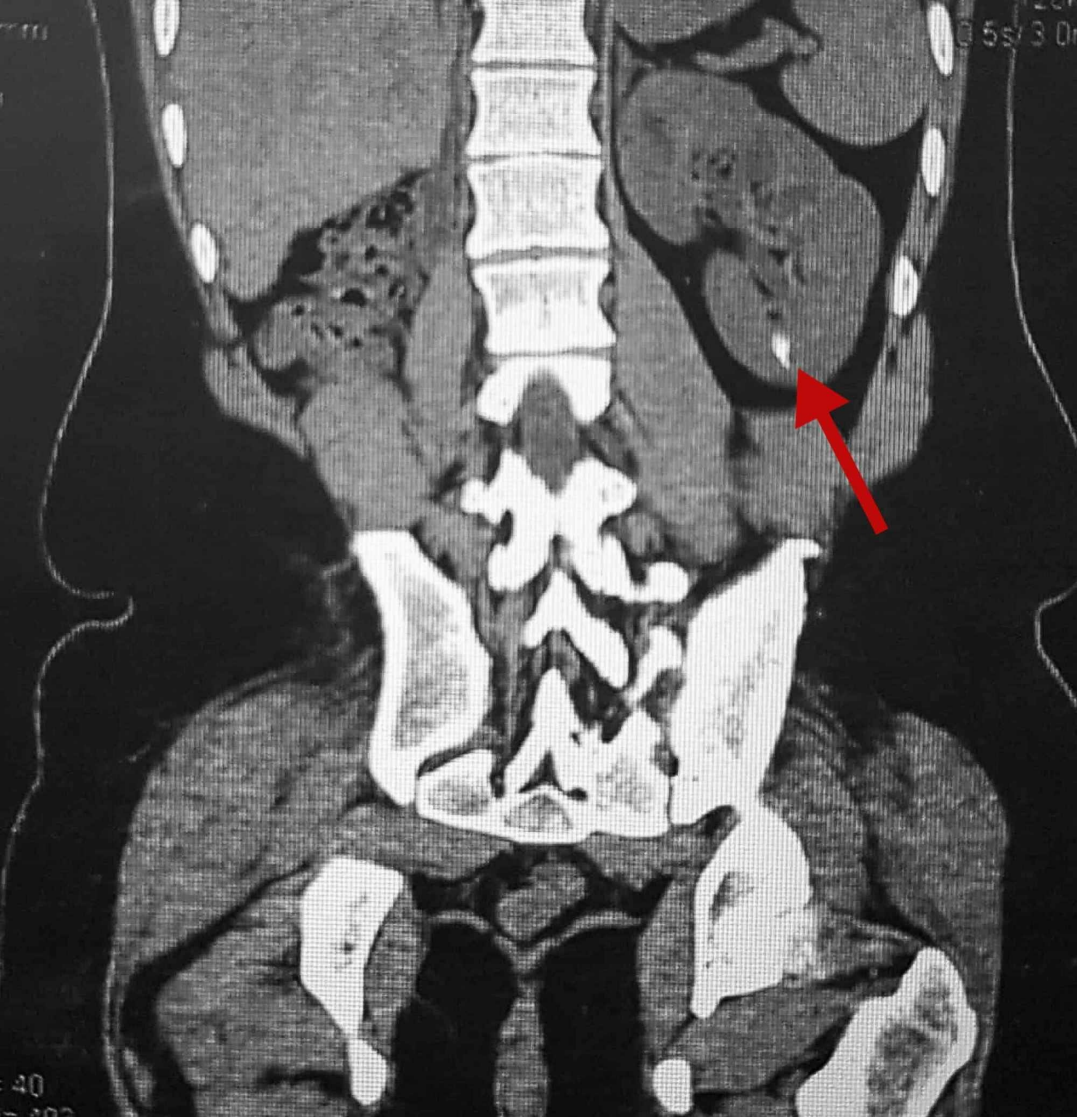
CT scan of the kidney, ureter and bladder showing a small calculus projecting over the lower pole calyx of the left kidney
